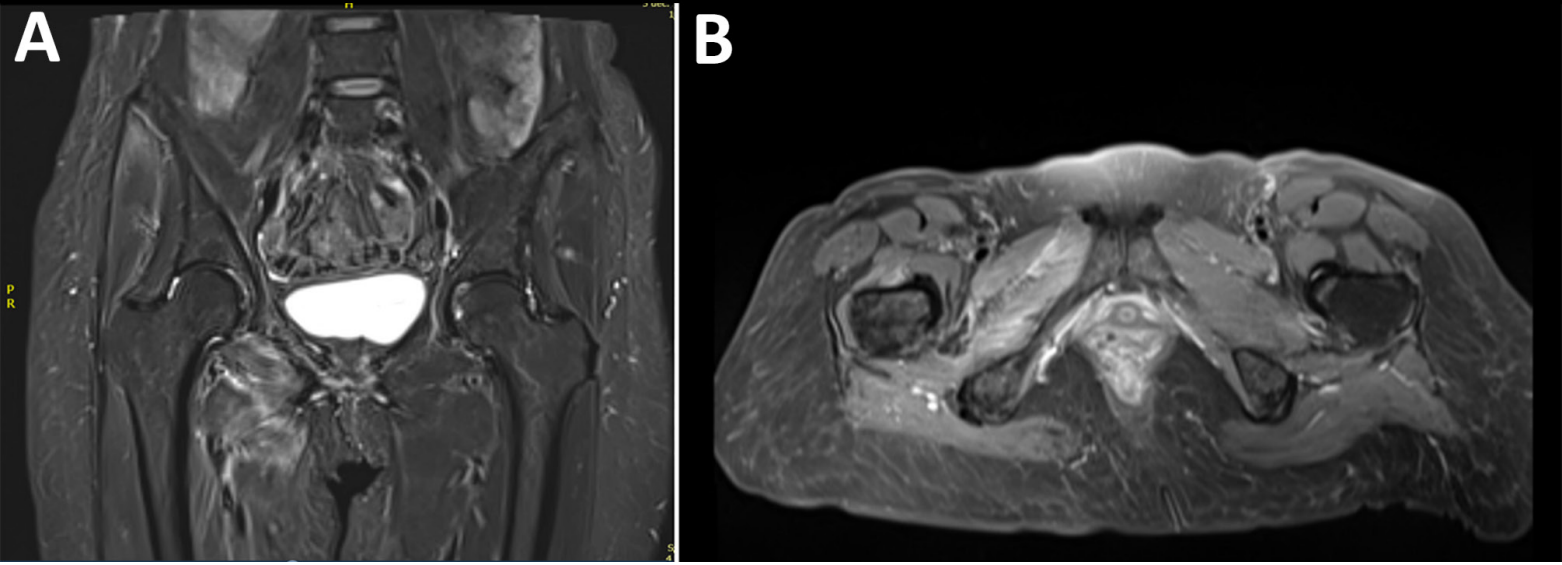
Coronal (A) and axial (B) T1 short-tau inversion recovery magnetic resonance imaging sequences of the hip in study of *Borrelia spielmanii*–associated neuroborreliosis in patient receiving rituximab, Belgium. Hyperintense infiltration of the right adductor muscle compartment (and partially of the hamstrings) was noted. No enhancement was seen after gadolinium injection.
